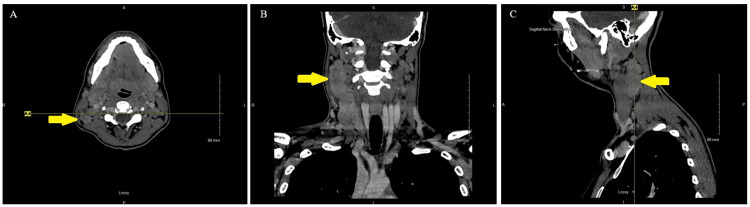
CT of the head and neck obtained at an outside hospital three days prior to admission Note: Shown are axial (Panel A), coronal (Panel B), and sagittal (Panel C) views of the cervical lymph mass. CT images were obtained with soft-tissue windows showing enlarged right level IIb/3 and 4a lymph nodes that extend craniocaudally subjacent to the sternocleidomastoid muscle. Most lymph nodes do not demonstrate normal fatty hilum. These findings increase concern for lymphoma over malignant lymphadenopathy or reactive adenopathy.
